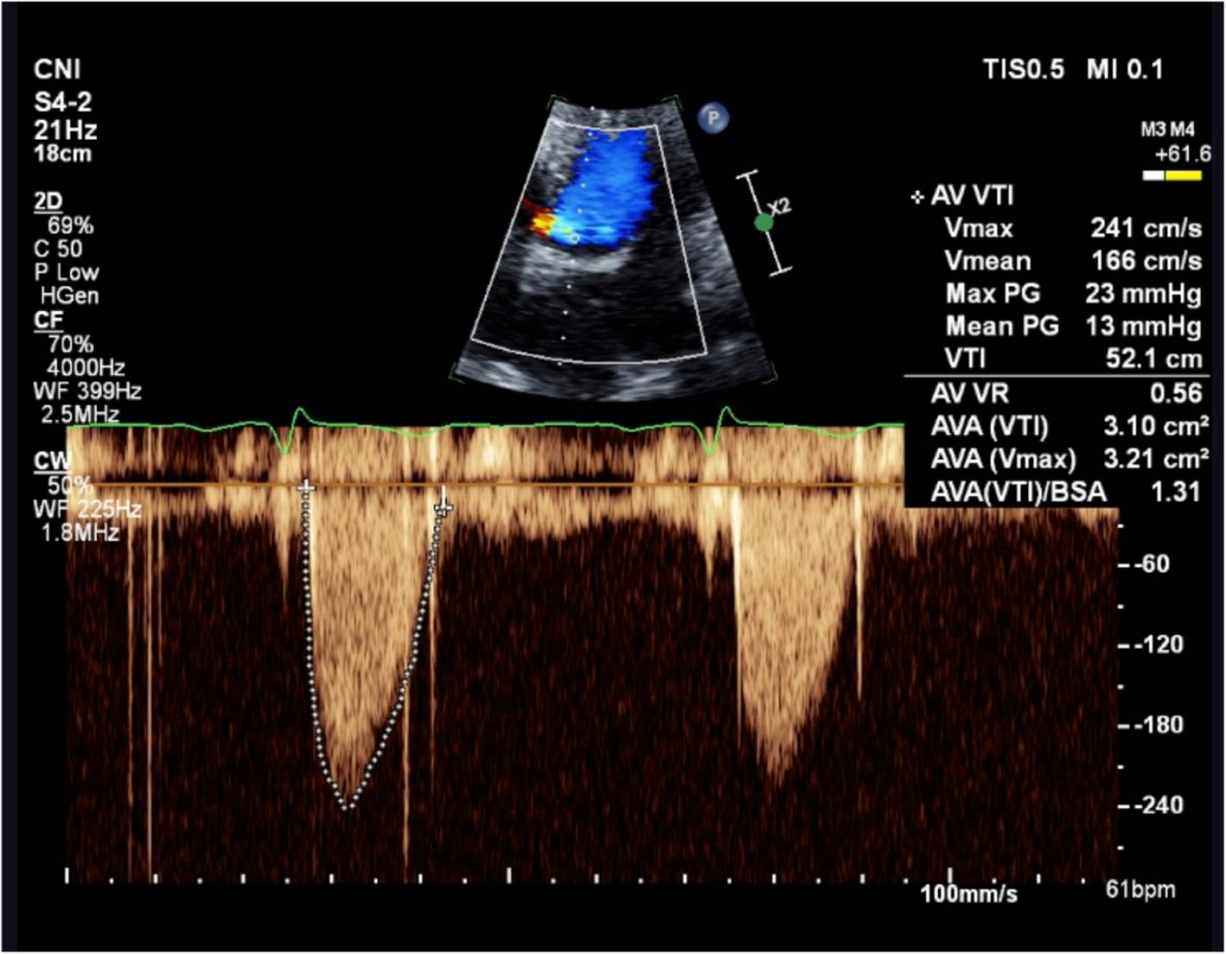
Transthoracic echocardiography after eight weeks of anticoagulation. Raised transaortic gradient returned to normal
